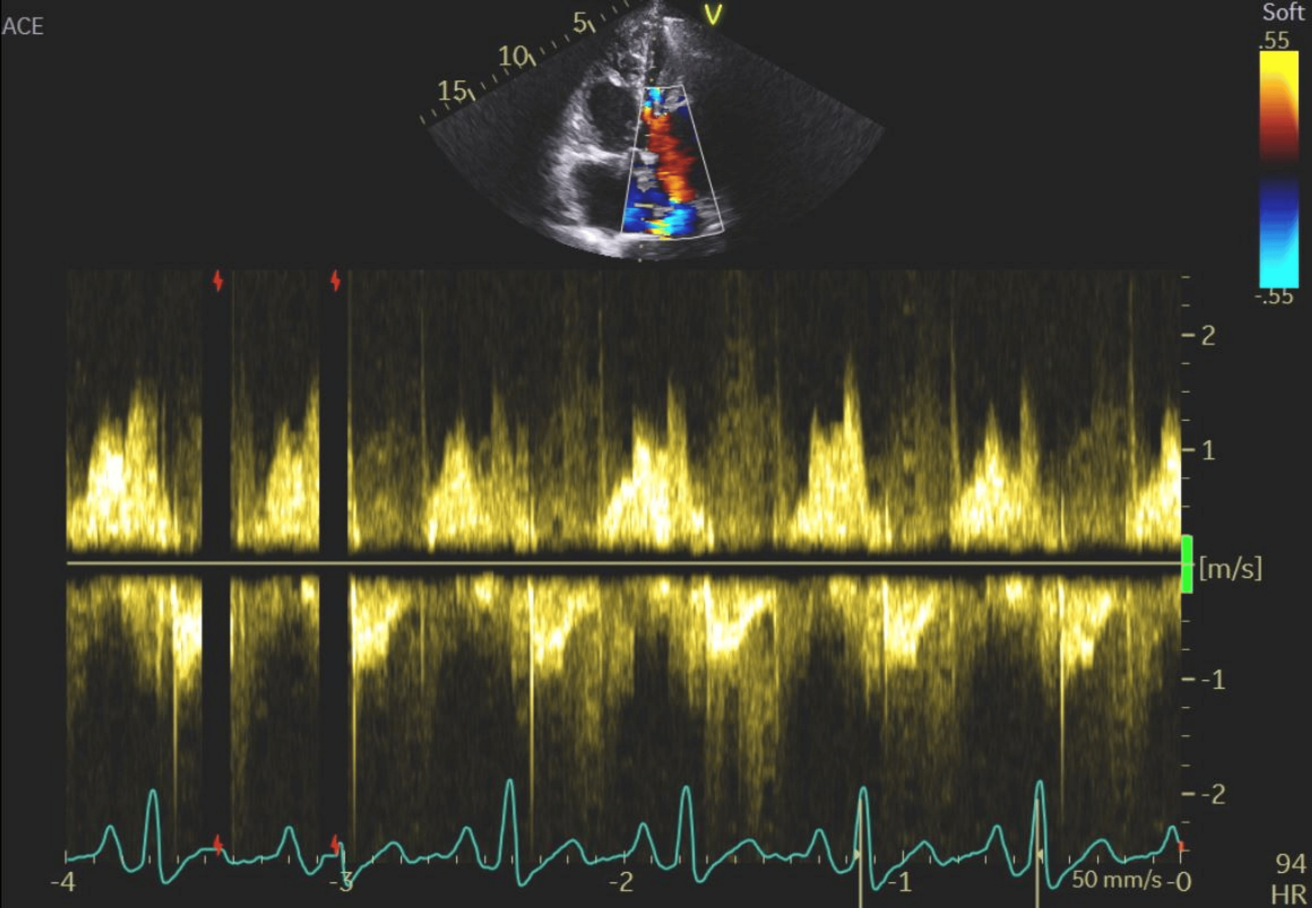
Spectral Doppler of flow across the mitral valve showing a biphasic flow with a dominant E-wave with a peak velocity of about 1.2 m/s and an A-wave of about 0.7 m/s, suggesting a normal or mildly increased diastolic gradient without features of significant left ventricular diastolic dysfunction.
